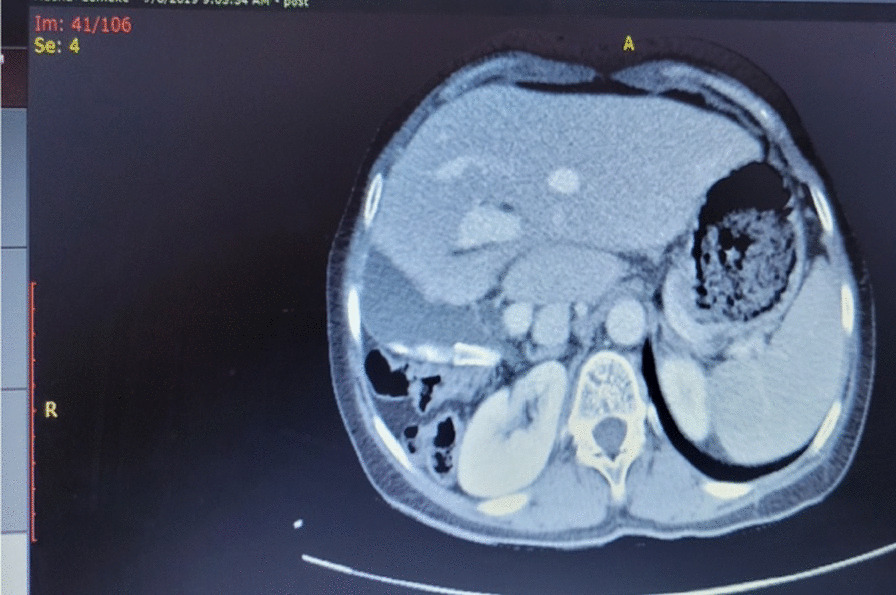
Abdominal computed tomography scan showing retrohepatic gallbladder and multiple radiopaque foci in the gallbladder colonic diaphragmatic interposition
